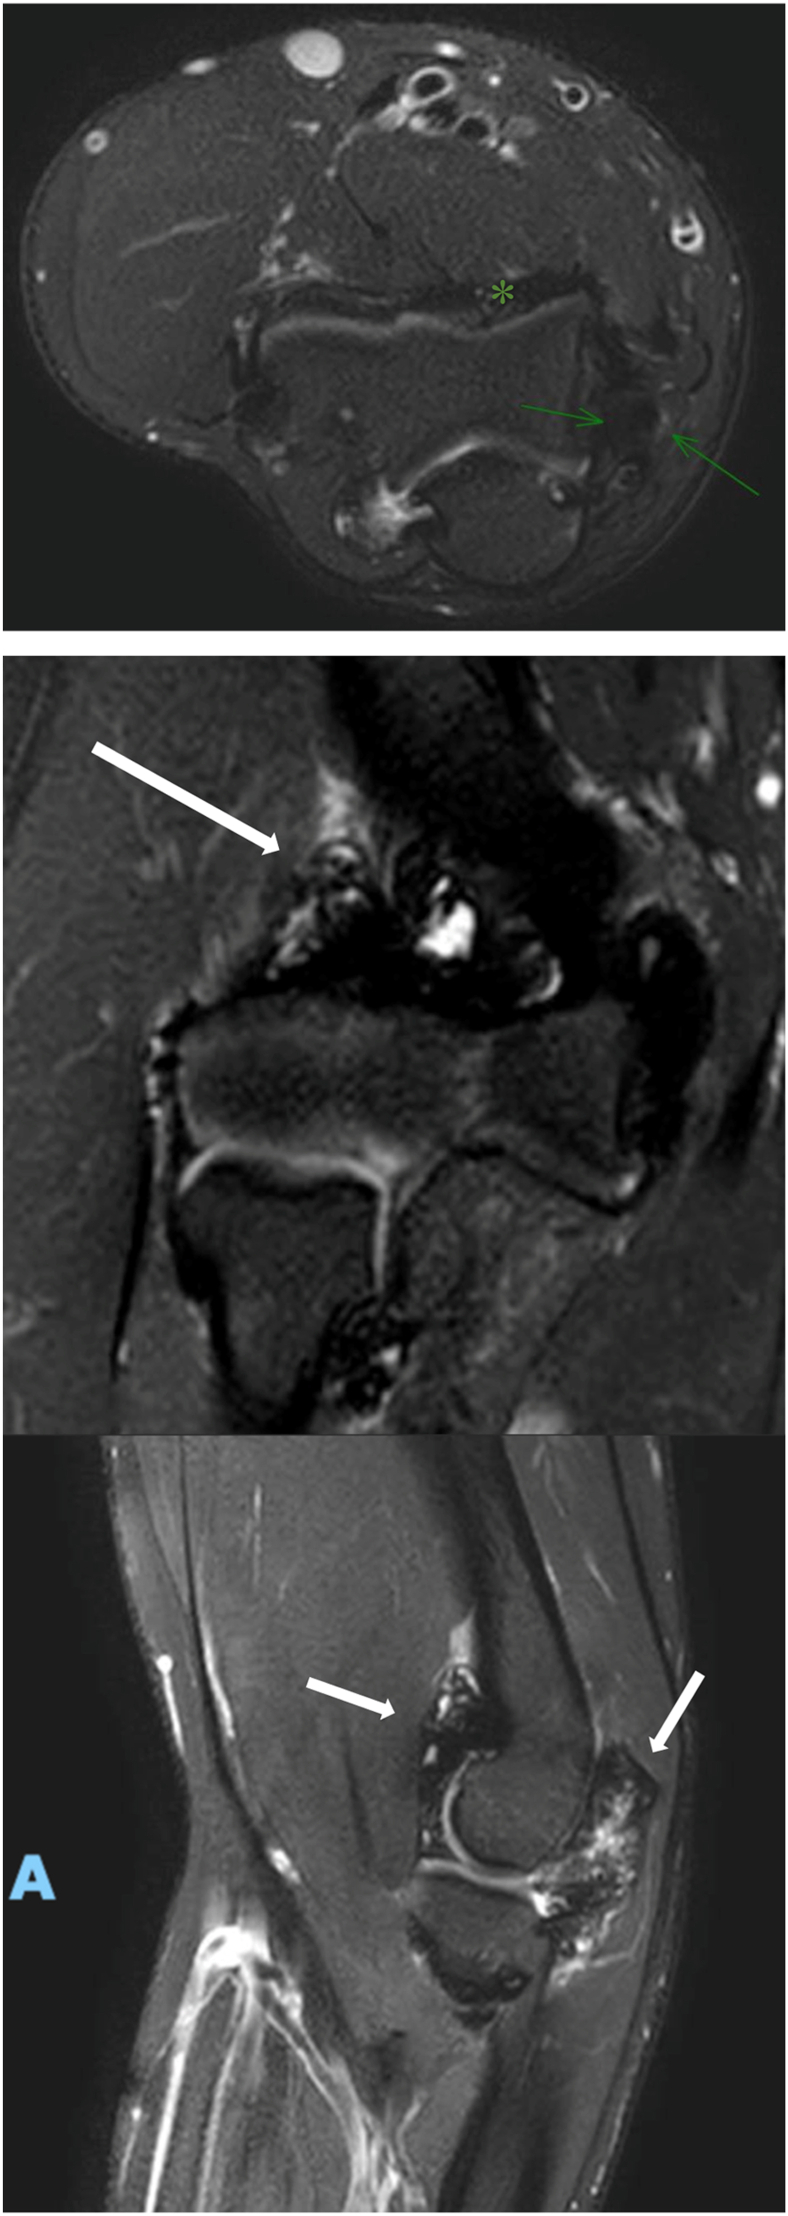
Preoperative MRI showing synovial thickening (∗), displacement of ulnar nerve (green arrows) and diffuse intra-articular nodular masses (white arrows). *MRI*, magnetic resonance imaging.
